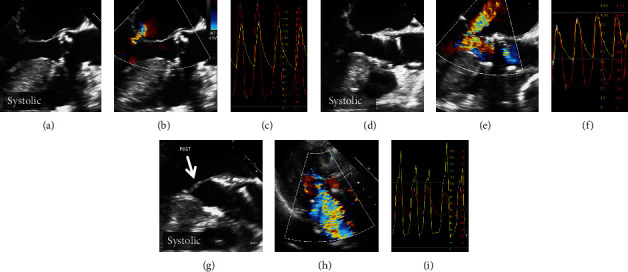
(a–c) No systolic anterior motion (SAM) was observed before transcatheter aortic valve implantation (TAVI). (d–f) Five minutes after TAVI, there was no SAM or pressure gradient. (g) Fifteen minutes later, SAM clearly emerged. (h) Transthoracic echocardiography showed severe mitral regurgitation. (i) Pressure gradient was >50 mmHg at the left ventricular outflow tract.
